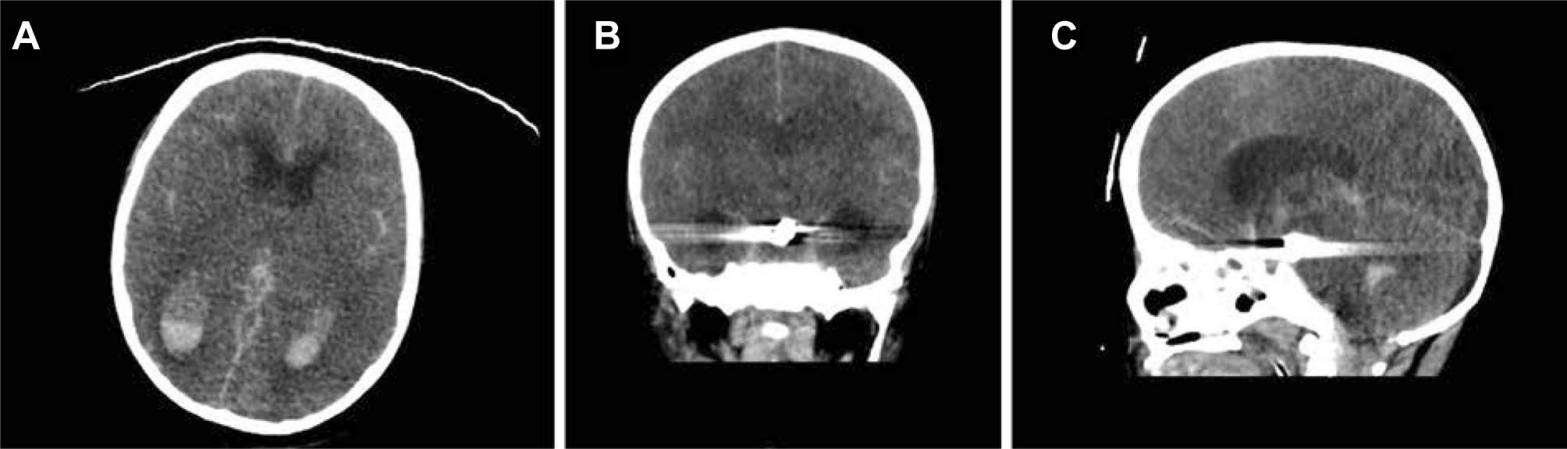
These are computerized tomography (CT) images after the sudden deterioration of the patient, showing intraventricular hemorrhage and further spread of the subarachnoid hemorrhage with hydrocephalus and significant edema (A–C)
